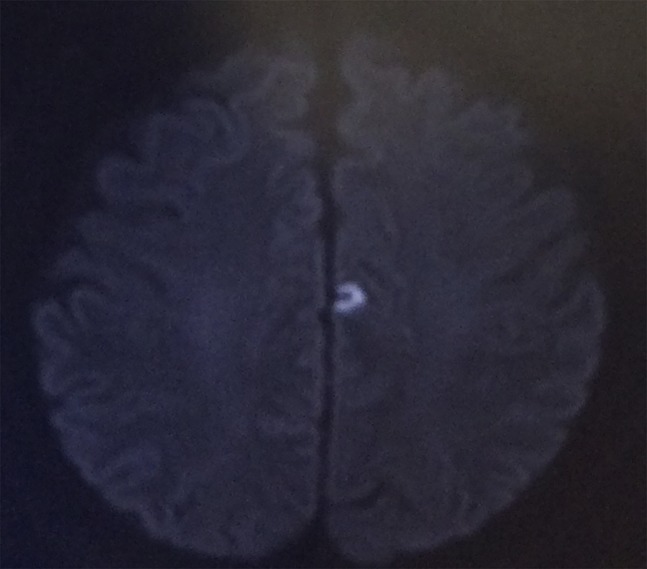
MRI diffusion image of the brain.
